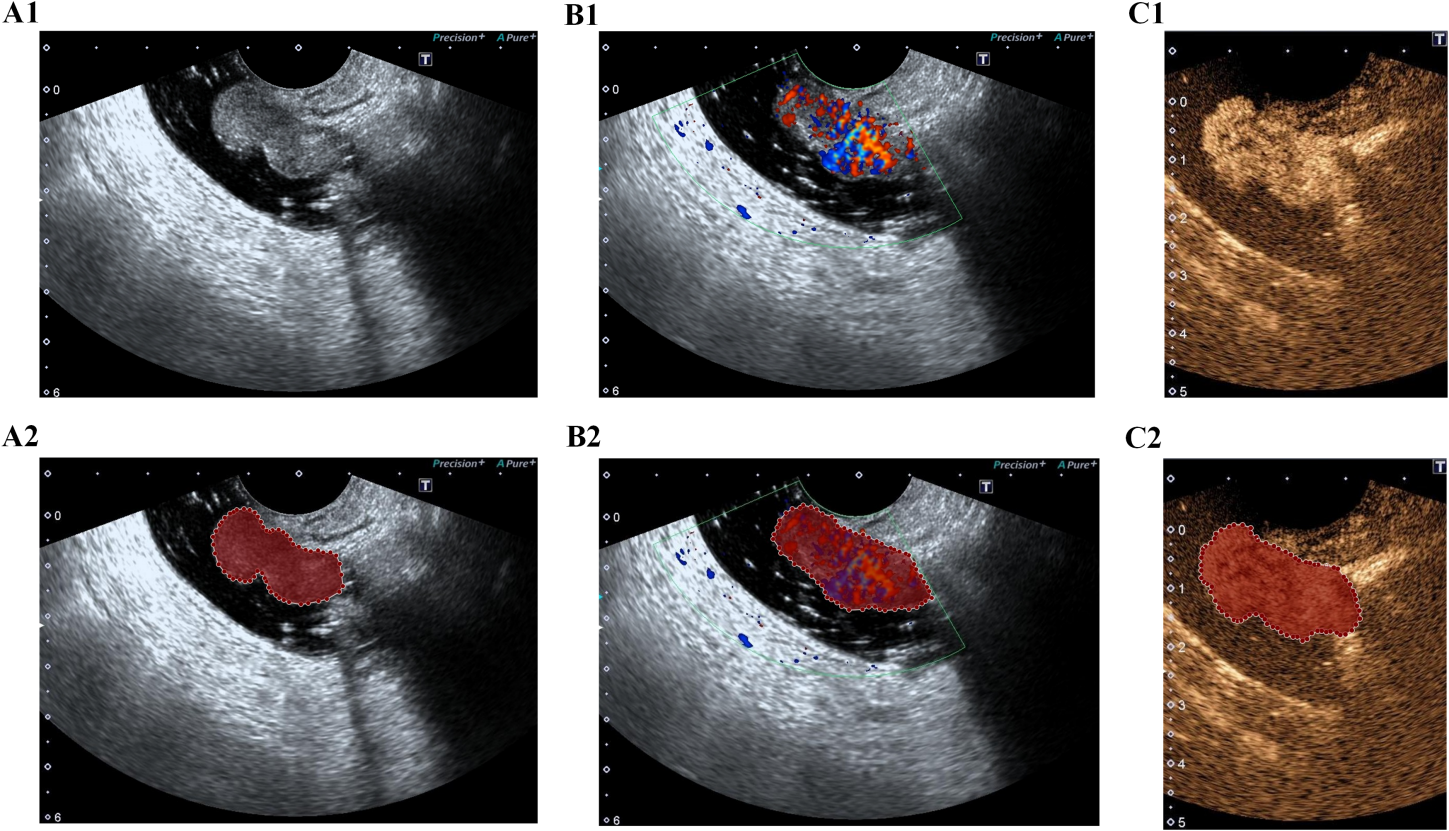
Representative examples of tumor segmentation on ultrasound images. (A) TDUS images before and after tumor segmentation. (B) CDUS images before and after segmentation. (C) CEUS images before and after segmentation.
